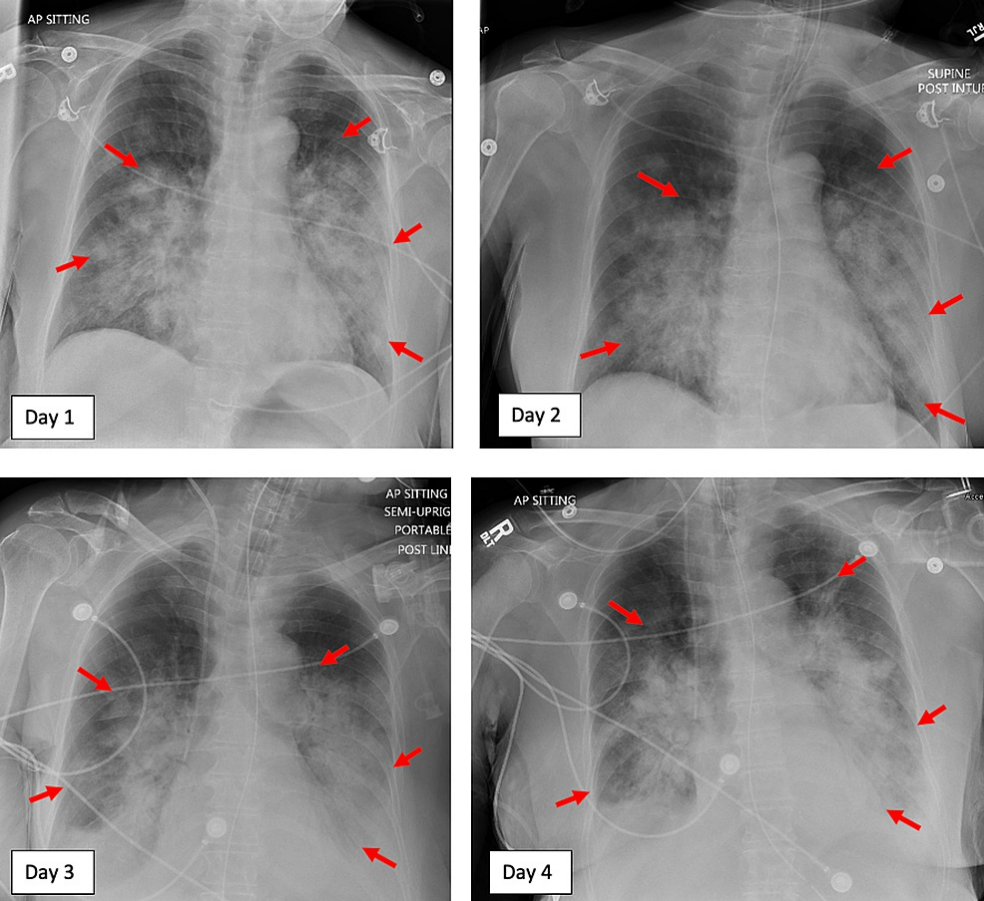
Sequential chest X-rays during the patient’s four-day hospitalization. The patient’s bilateral pulmonary infiltrates expanded over the four-day period. Her bilateral pleural effusions are marked with red arrows.
